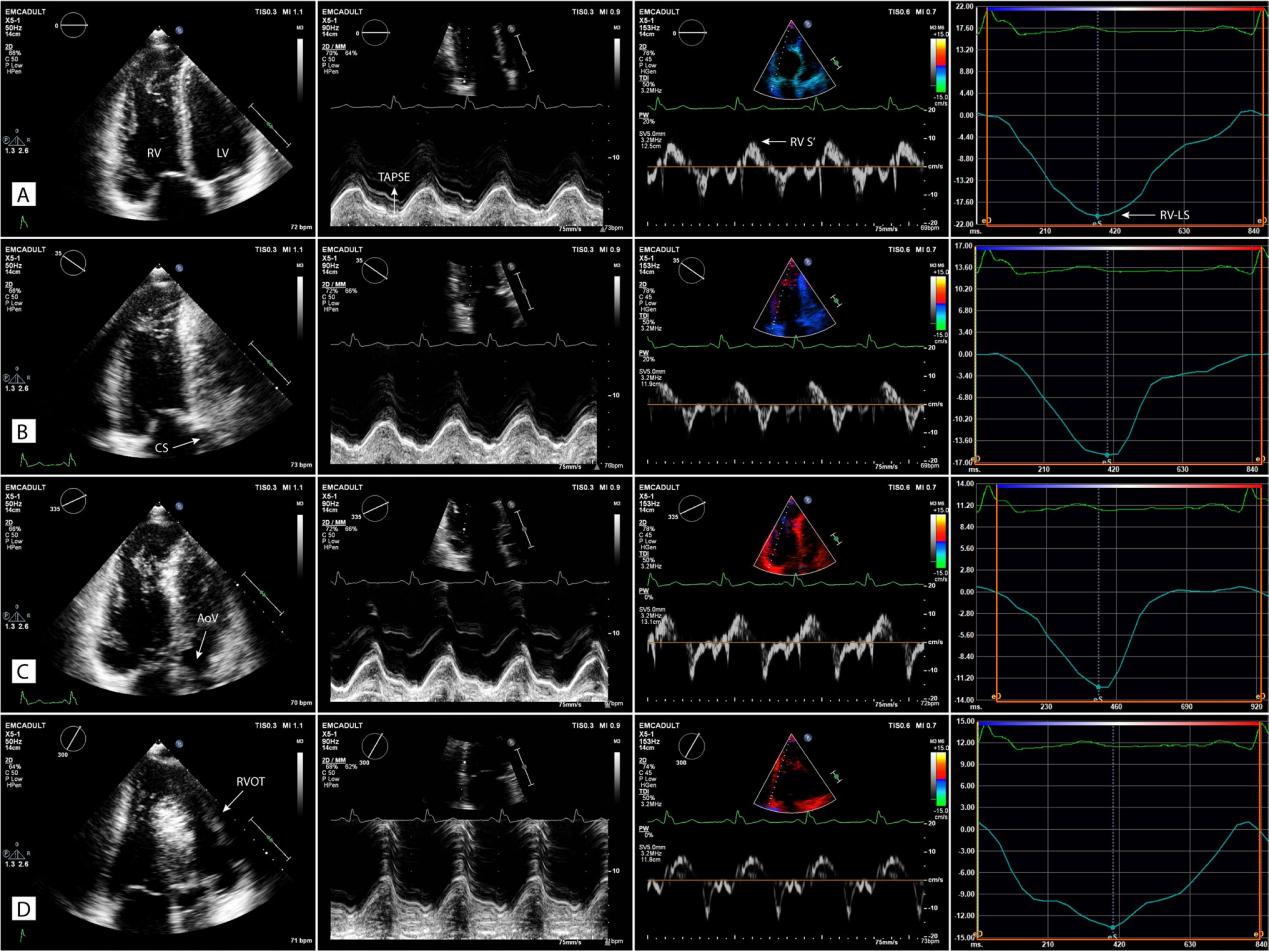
Echocardiographic images of the four multi-plane right ventricular (RV) views (A-D) with corresponding quantitative functional parameters of the respective RV walls (L-R panels). A Focused four chamber view (0°), lateral wall; B coronary sinus view (+ 40°), anterior wall; C aortic view (− 40°), inferior wall; D coronal view (− 90º), inferior wall and RVOT anterior wall. Second panel (centre left): tricuspid annular plane systolic excursion (TAPSE); third panel, (centre right): tricuspid annular peak systolic velocity (RV-S′); fourth panel (far right): RV wall longitudinal strain (RV-LS). *LV* left ventricle, *CS* coronary sinus; *AoV* aortic valve, *RVOT* right ventricular outflow tract
